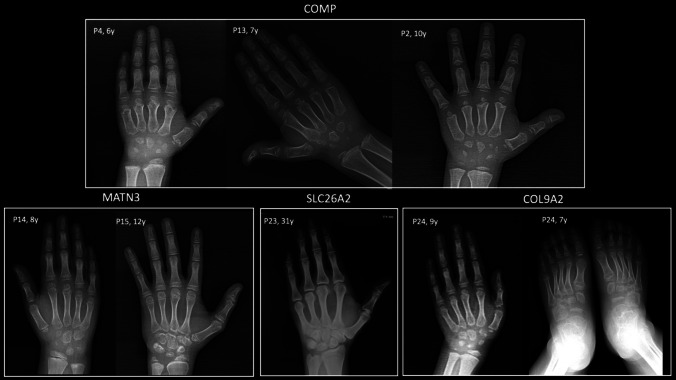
Hand radiographs of the patients and the foot radiograph of P24. All patients, except for the adult patient (P23), exhibit small and irregularly shaped carpal bones and epiphyses of the distal radius and ulna. Patients with *COMP* variants have short and broad metacarpal and phalangeal bones (brachydactyly), characterized by small, irregularly shaped epiphyses and metaphyseal irregularity. Patients with *MATN3* variants do not exhibit brachydactyly but also show mild contour irregularity of the carpal bones. Irregularly shaped tarsal bones are observed in P24, who has a *COL9A2* variant. P, patient; y, years
